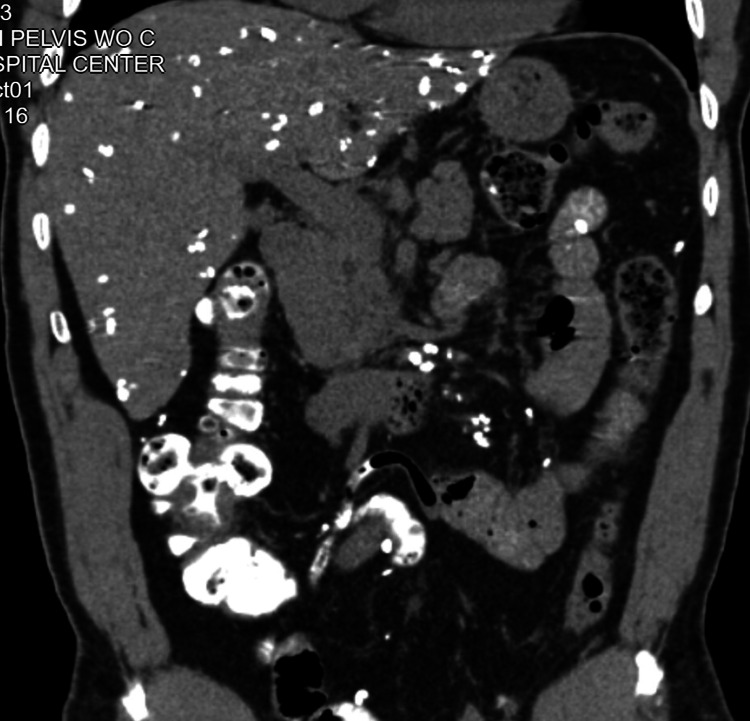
CT demonstrating radiodensities throughout the abdomen and mesentery CT: Computed tomography
